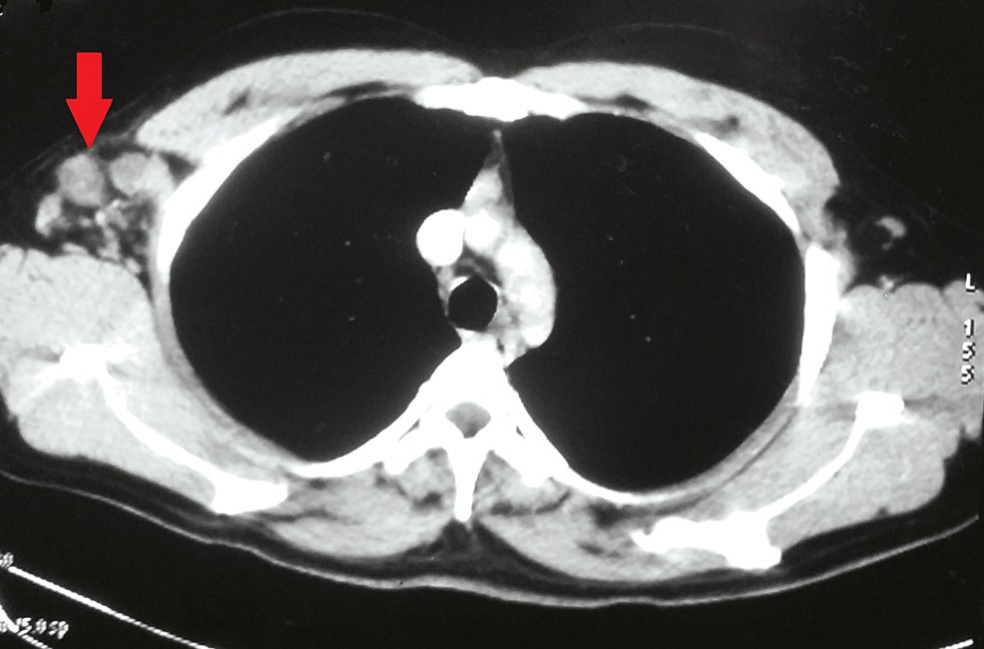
A contrast-enhanced computed tomography of the chest suggestive of multiple enlarged discrete necrotic lymph nodes in the right axillary region.
